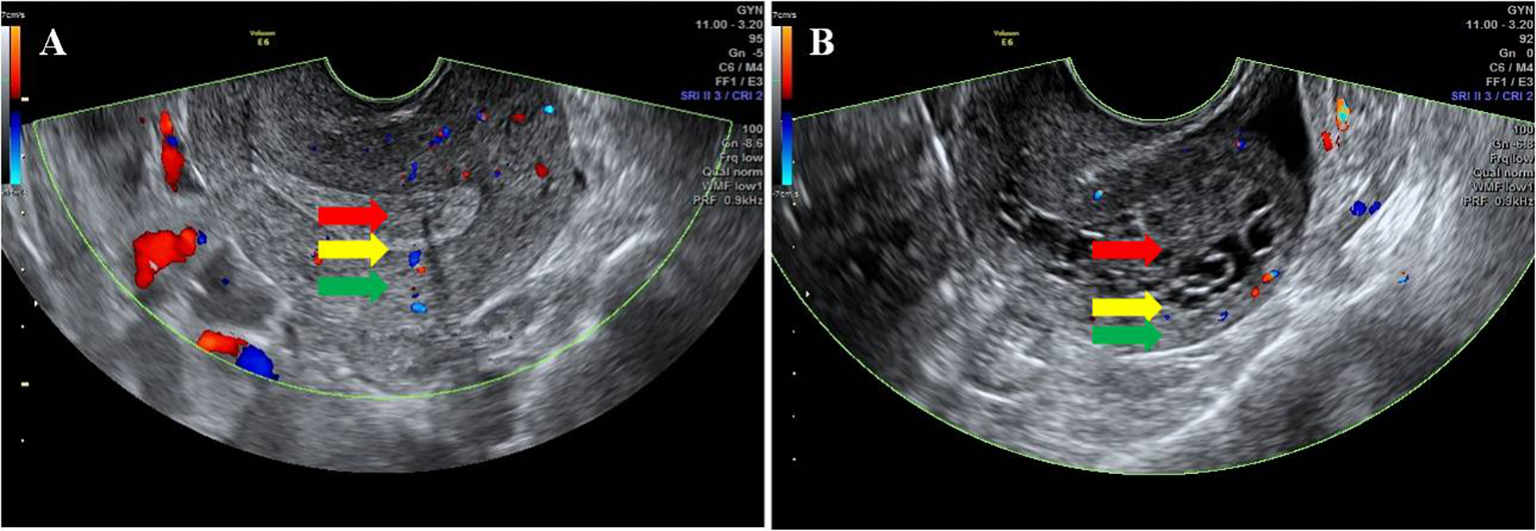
Ultrasonic characteristics of the EMJ. The red arrow indicates the endometrium, the green arrow indicates the myometrium, and the yellow arrow indicates the EMJ. (A) Clear EMJ. (B) Unclear EMJ.
